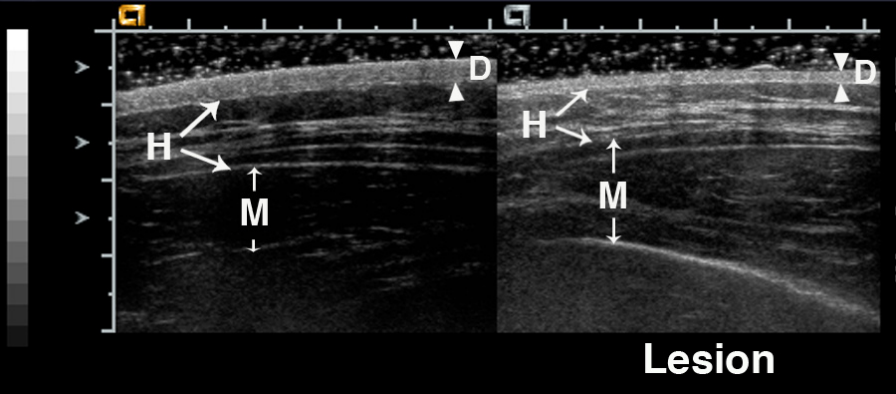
17 year old girl with generalized morphea that began over 13 years earlier. She has lesions on her back, abdomen, arms, and leg. Most lesions show dyspigmentation and mild tissue loss, but her back lesions have increased warmth. Ultrasound of a back lesion shows thinning of the dermis and hypodermis, and an increase in lesion dermal echogenicity (dermis echogenicity = 1). There are highly echogenic horizontal bands in the hypodermis layer of both the normal and lesion hypodermis layers. The echogenicity of these bands is increased in the lesion, as is the base level of hypodermal echogenicity (hypodermis echogenicity = 2). The lesion muscle shows a patchy increase in echogenicity compared to the normal muscle (deep tissue echogenicity = 1). Arrowheads indicate boundaries of dermis, while arrows indicate boundaries of hypodermis and muscle.
